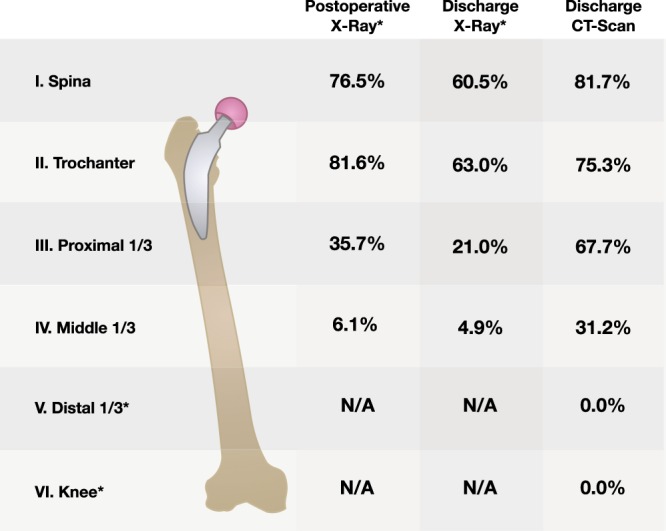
Air entrapment. Frequency (in %) of air entrapment in x-rays taken directly postoperatively and x-rays as well as CT-scans taken at discharge. *X-rays did only cover the region up to the middle 1/3 of femur, whilst the CT-scan covered all regions.
